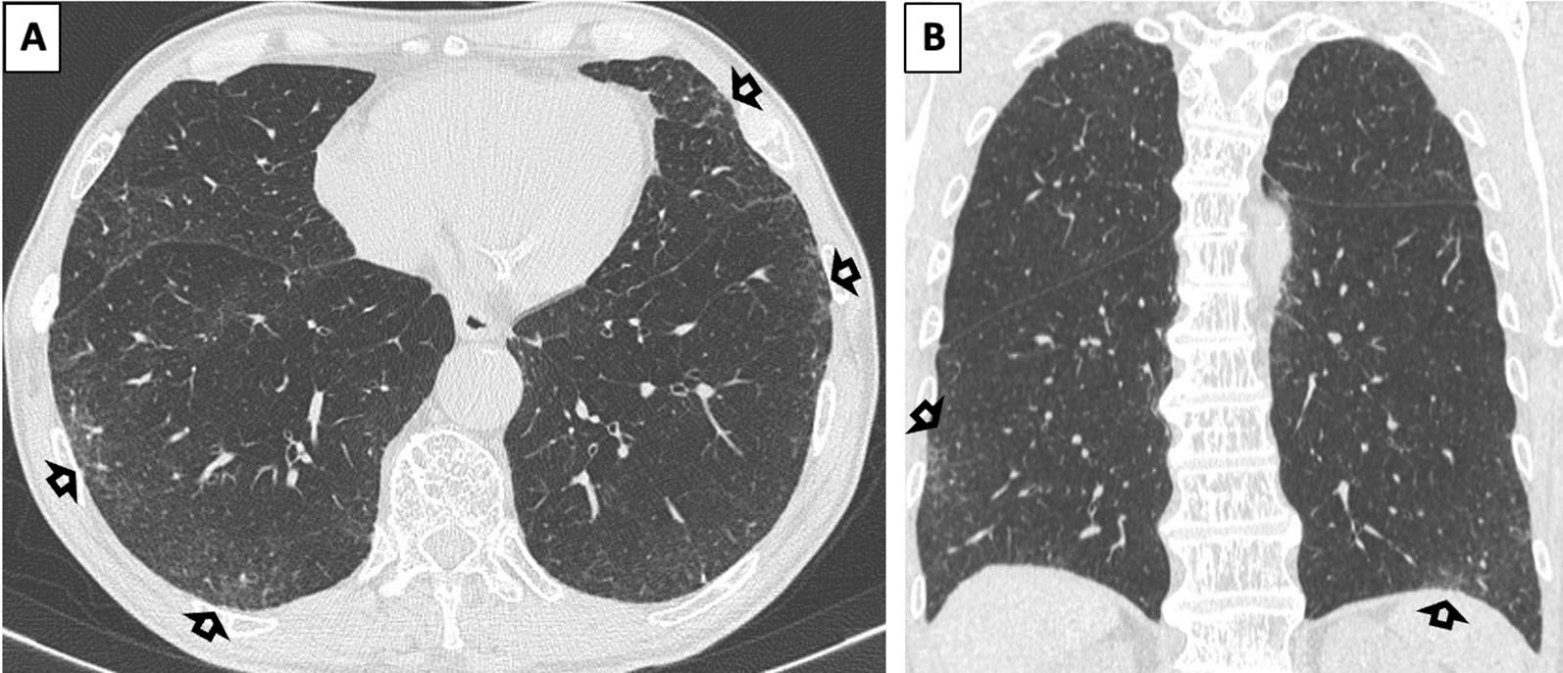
a, b Axial (a) and coronal (b) CT images without contrast (slice thickness of 1 mm) of a 79-year-old gentleman display bilateral GGOs and reticular opacities (*open arrows*) in the subpleural regions of both upper and lower lobes, compatible with non-fibrotic subpleural ILAs
